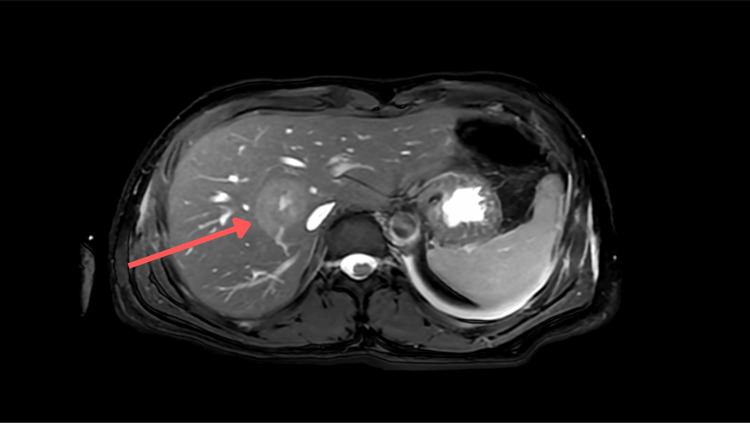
CT image of the liver showing metastases. The red arrow points at liver metastases. CT: computed tomography
